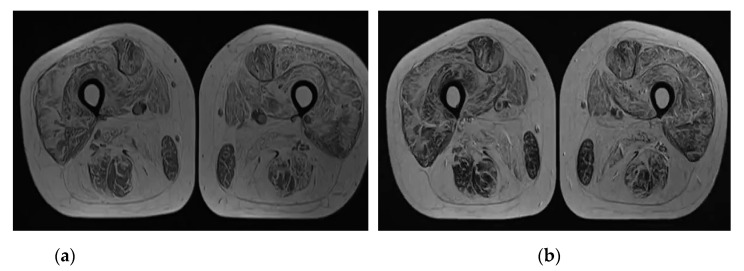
(a) Axial T1-weighted MRI scan with diffuse increase of signal intensity from all muscles of the both shins; (b) Axial T2 weighted MRI scan with diffuse increase of signal intensity from all muscles of the both shins.
